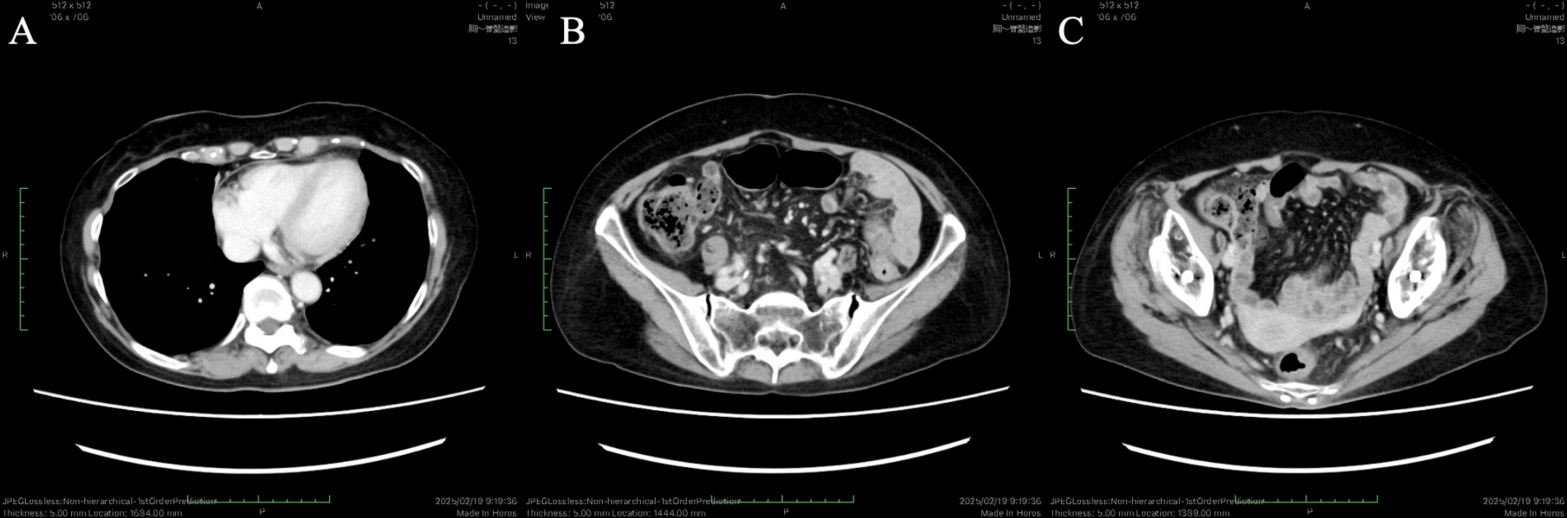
CT images obtained after six cycles of treatment with paclitaxel, carboplatin, and bevacizumab Follow-up chest and abdominal CT demonstrates complete resolution of the pericardial and pleural effusions, peritoneal dissemination, and ascites, corresponding to a complete response according to the RECIST criteria. RECIST, Response Evaluation Criteria in Solid Tumors
